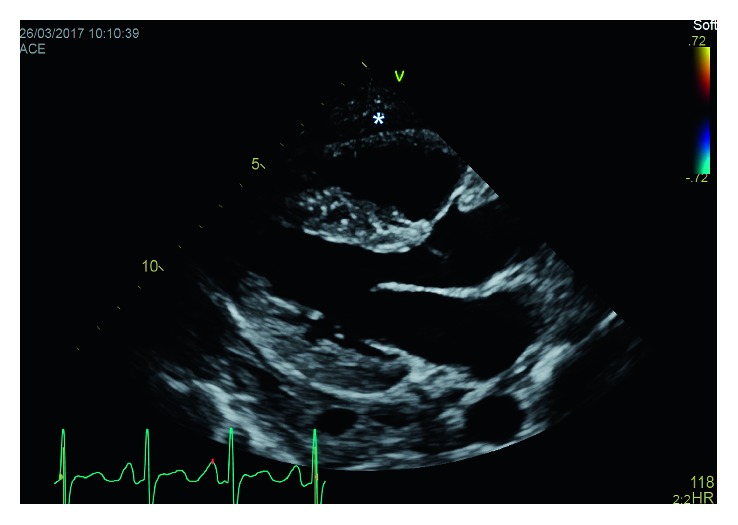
Transthoracic echocardiogram parasternal long-axis view (pericardial effusion denoted by ∗).
